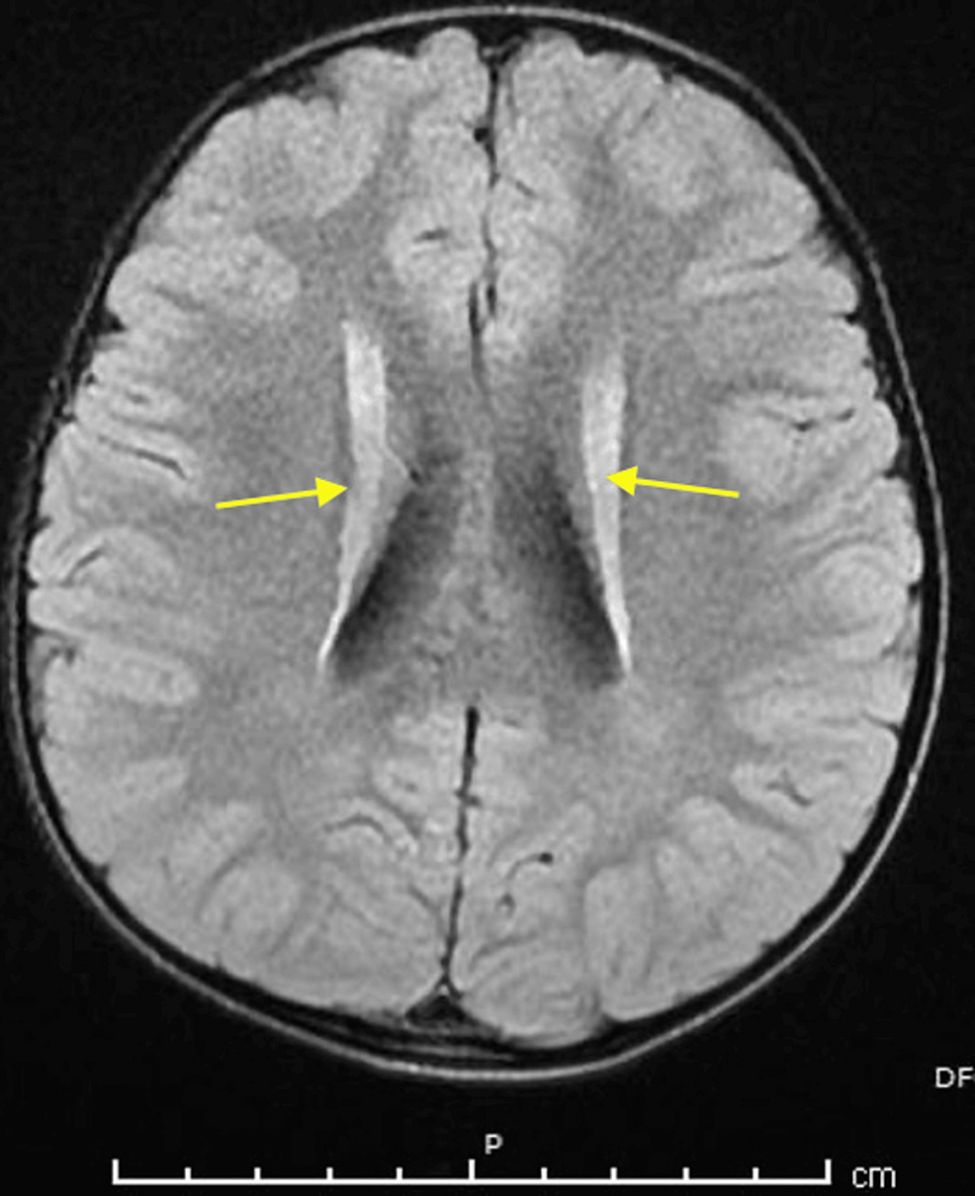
Magnetic resonance imaging of the patient's brain Chronic small vessel ischemia involving the periventricular white matter (Fazekas scale grade 1).
